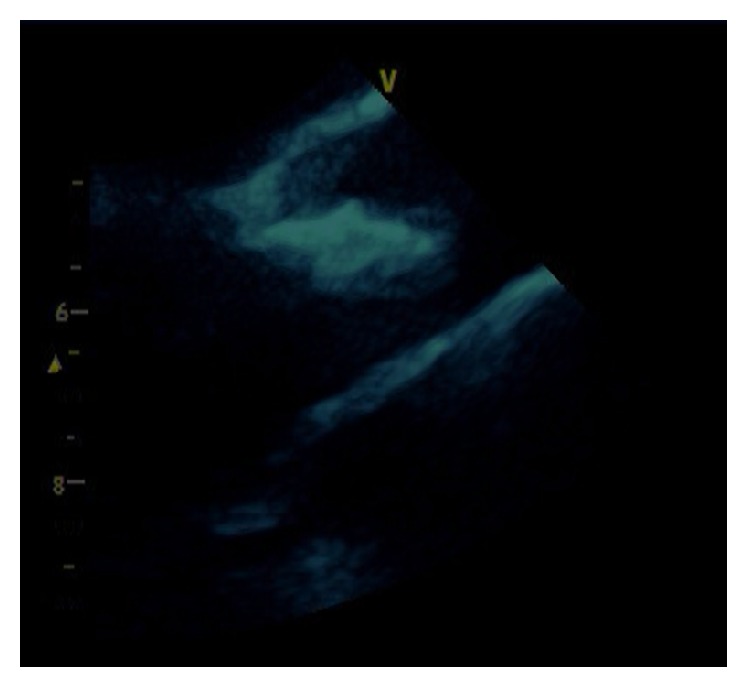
Aortic valve vegetation in parasternal view.
